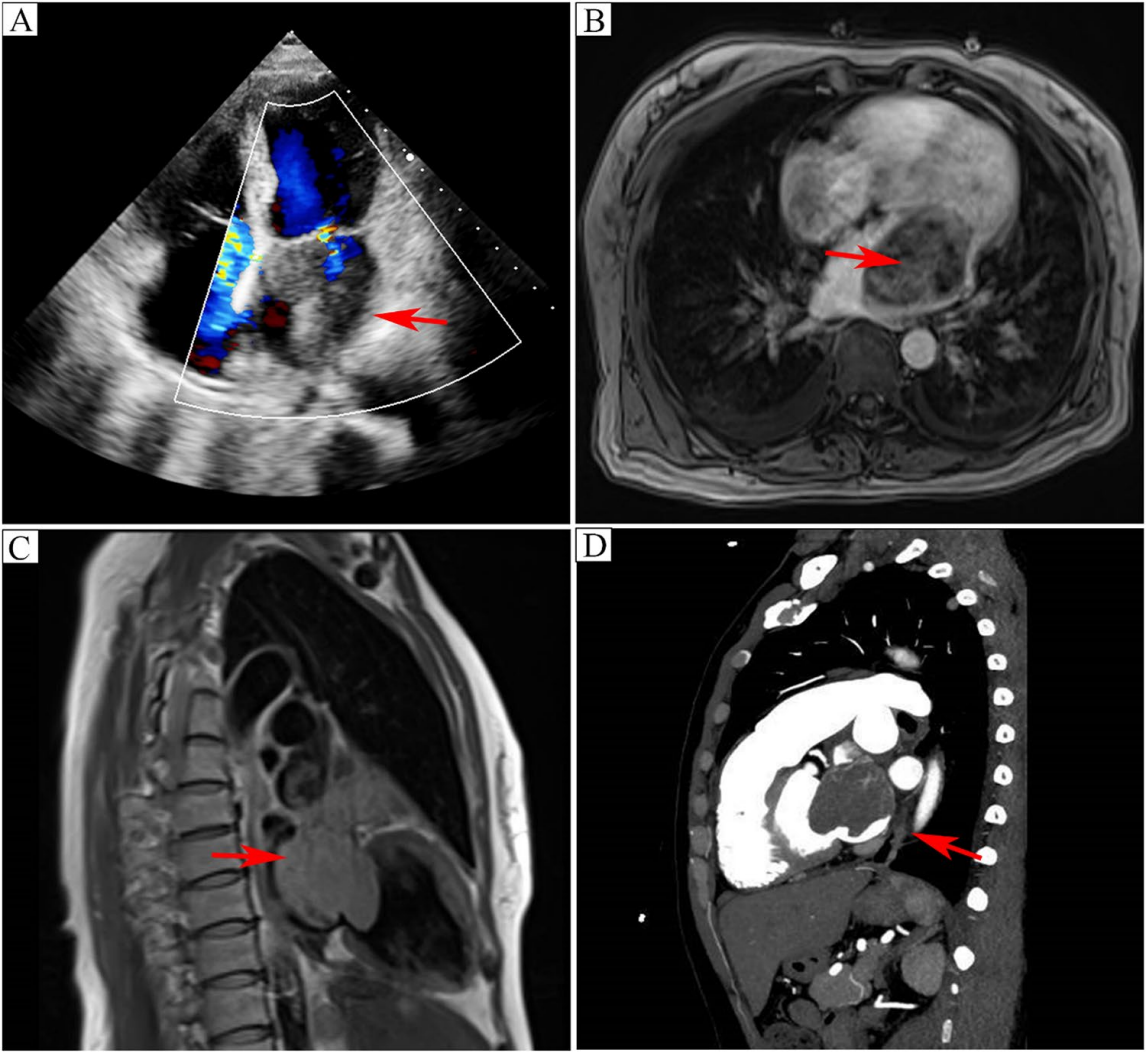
Transthoracic echocardiography, cardiac MRI, and CT angiography. A Transthoracic echocardiography captured the left atrial giant sarcoma, exhibiting a slightly weak echo and measuring 4.8 × 6.7 cm. B, C Transverse and sagittal cardiac MRI revealed a protruding and compressive left atrial mass. D Sagittal cardiac angiography demonstrated an irregular mass within the left atrium, locally extending into the left atrial appendage, oscillating in accordance with the cardiac cycle, displaying high activity and notable enhancement
